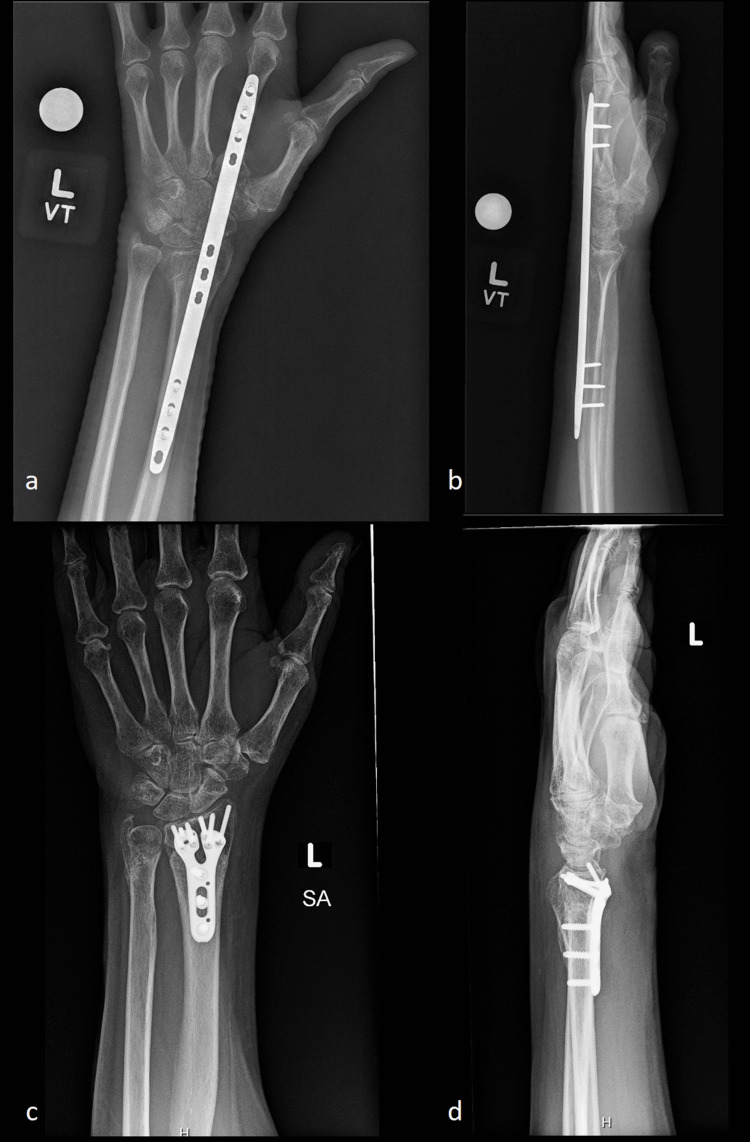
Post-operative distal radius fracture radiographs Post-operative radiographs of dorsal bridge plate fixation (a, b) and volar locking plate fixation (c, d)
